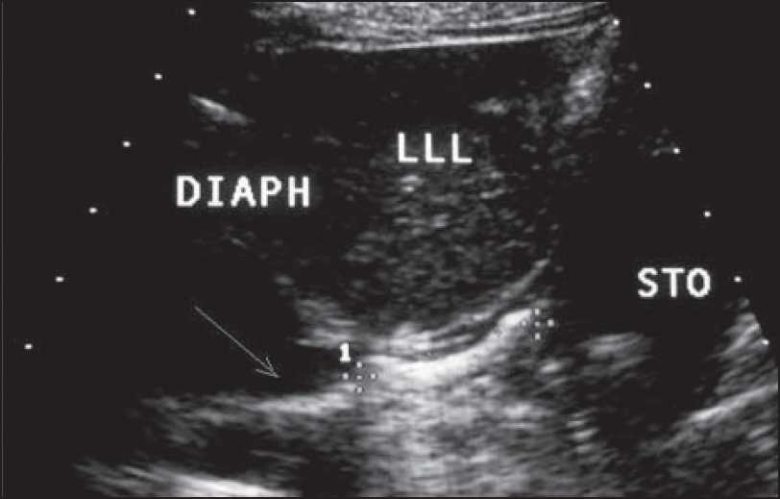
Retrograde flow of gastric contents through the lower esophagus detected by sonography (arrow)
